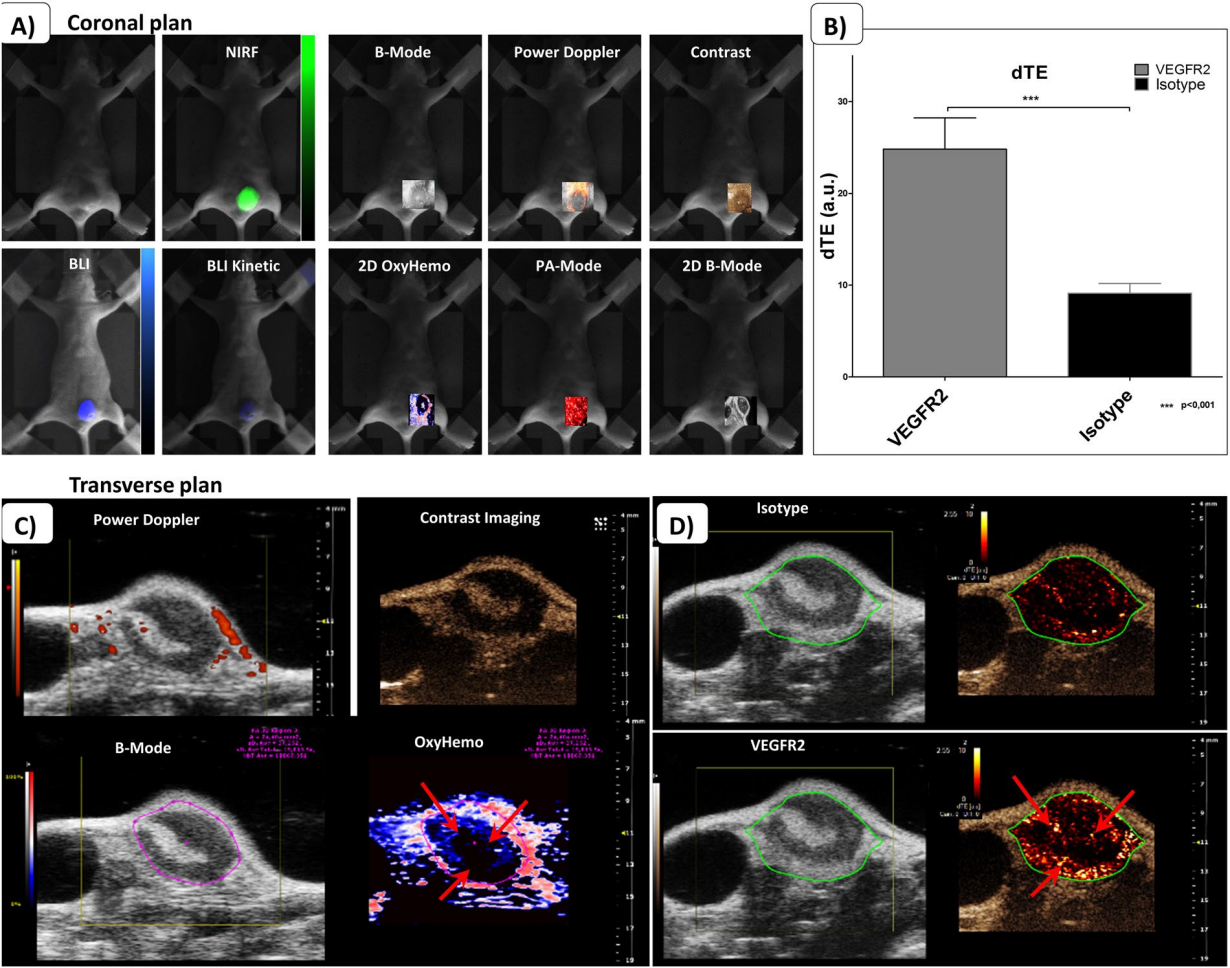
Comparisons of modalities: From biophotonics to ultrasounds and photoacoustics. (A) 2 dimensions biophotonics acquisitions (BLI, BLI kinetic and NIRF) are compared to 3D acquisitions from the VEVO system. Here are presented 2D coronal rendering from 3D acquisitions acquired thanks to the stepper motor. Acquisitions for B-Mode, Power Doppler, Contrast, Oxy-Hemo and PA-Mode can easily be merged on black and white image from the camera. (B) Assessment of the differential Targeted Enhancement (dTE), following the destruction of both targeted and non-targeted microbubbles. A higher dTE means a greater microbubbles fixation, so an important VEGRF2 expression. (C) Examples of comparisons that can be performed on the same slice from the same tumor *in vivo*. In this set of images, we find transverse Power Doppler, Contrast Imaging and Photoacoustic OxyHemo-Mode. Red arrows highlight hypoxic areas corresponding to VEGFR2 expression. (D) Corresponding transverse parametric images from ultrasound molecular imaging showing the VEGFR2 expression as compared signals from microbubbles targeted via an isotype antibody.
